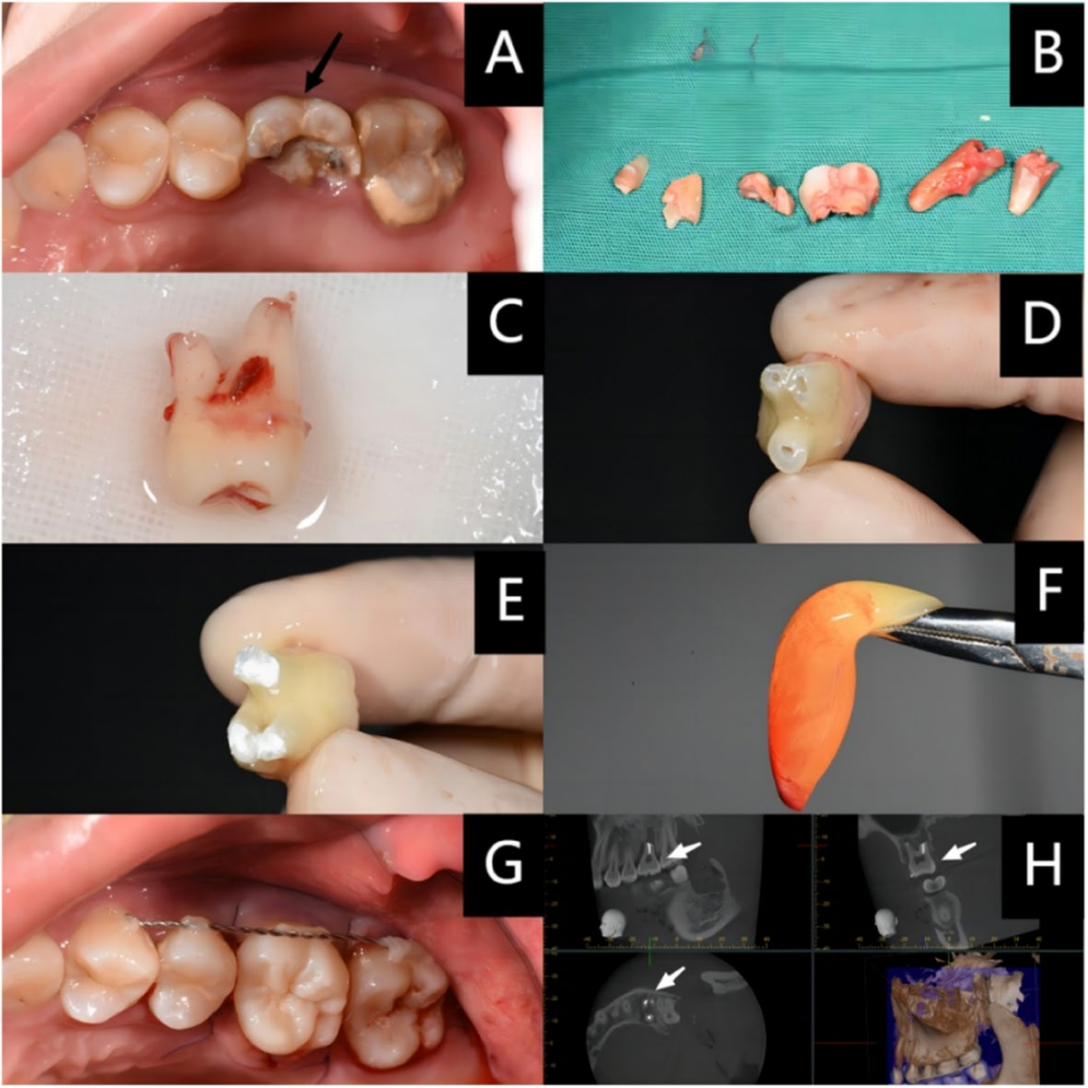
Residual crown in maxillary posterior region (A); Extraction of residual crown (B); Donor tooth with partially broken root (C); Apical trimming and retrograde preparation (D); IRoot BP plus filling (E); CGF for placement of alveolar socket to promote healing (F); Fixation with elastic ligature wire and flowable resin (G); CBCT scan was taken immediately after the procedure (H)
